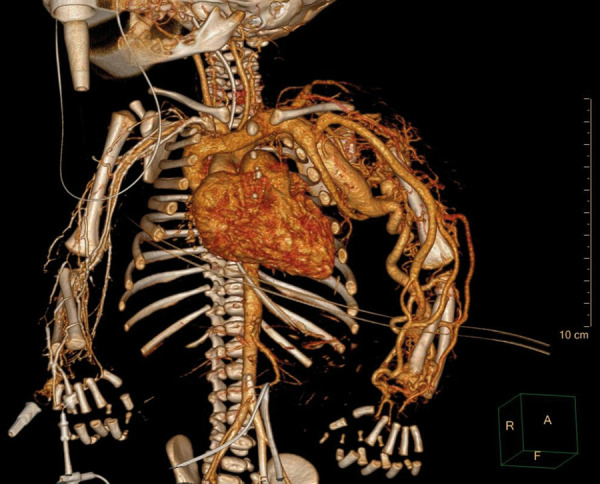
CT angiography showing AV malformations in the left scapula and shoulder region and AV fistulas between *a. subclavia sin.* and *v. brachiocephalica sin*.
